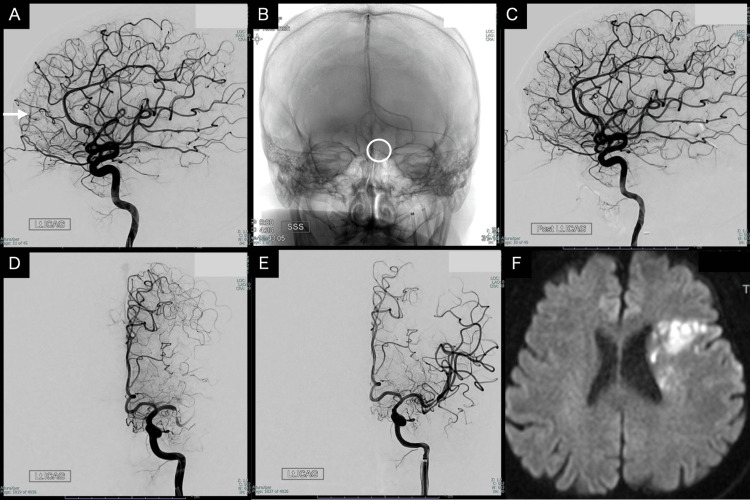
TVE of the first patient (A) Preoperative left ICAG. The white arrow indicates the draining vein. (B) Microcatheter positioned at the foot of the draining vein near the shunt point (centered within the white circle). (C) Postoperative left ICAG demonstrating the complete obliteration of the shunt. (D) Intraoperative occlusion of the left M1 segment. (E) Recanalization of the same vessel following mechanical thrombectomy. (F) Postoperative MRI (DWI) demonstrating an infarct in the left frontal lobe. TVE: transvenous embolization; ICAG: internal carotid angiography; MRI: magnetic resonance imaging; DWI: diffusion-weighted imaging
